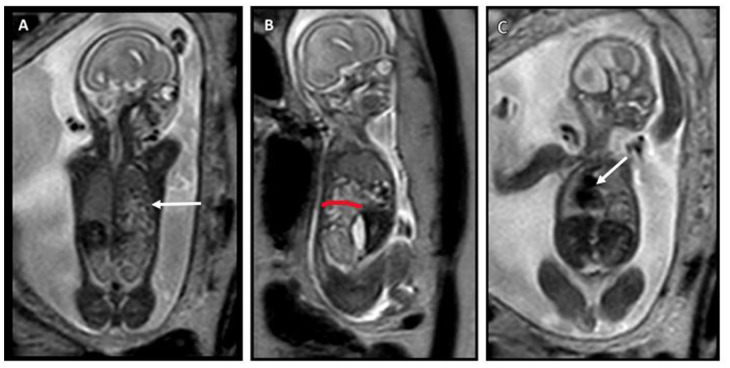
T2-weighted MRI images. (A) A coronal image at the posterior level near the vertebra, showing the left diaphragmatic defect and fluid-filled structures in the thorax (arrow), consistent with bowel loops. (B) A left sagittal image, more clearly depicting the posterior diaphragmatic defect (red line) and bowel in the thoracic cavity. (C) A more anterior coronal image, displaying the rightward shift of the heart and mediastinum (arrow).
